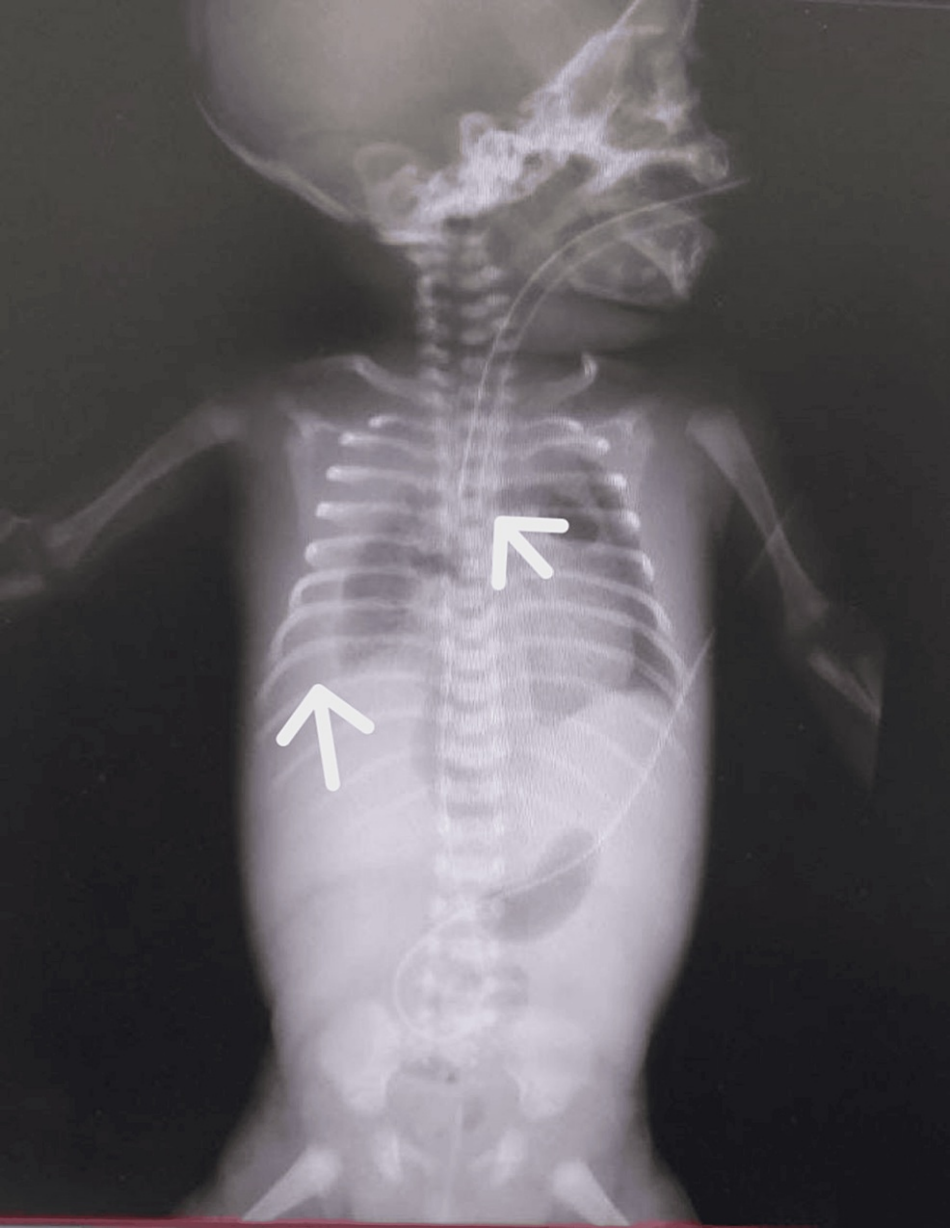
X-ray showing a right-side pleural effusion with an endotracheal tube in the site
